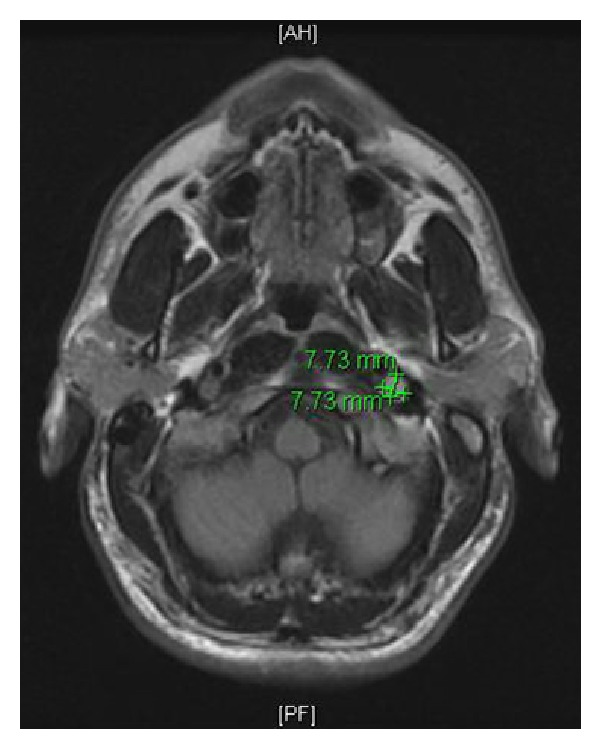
Axial Flair brain MRI demonstrating a mass in the left posterior carotid space at the skull base.
